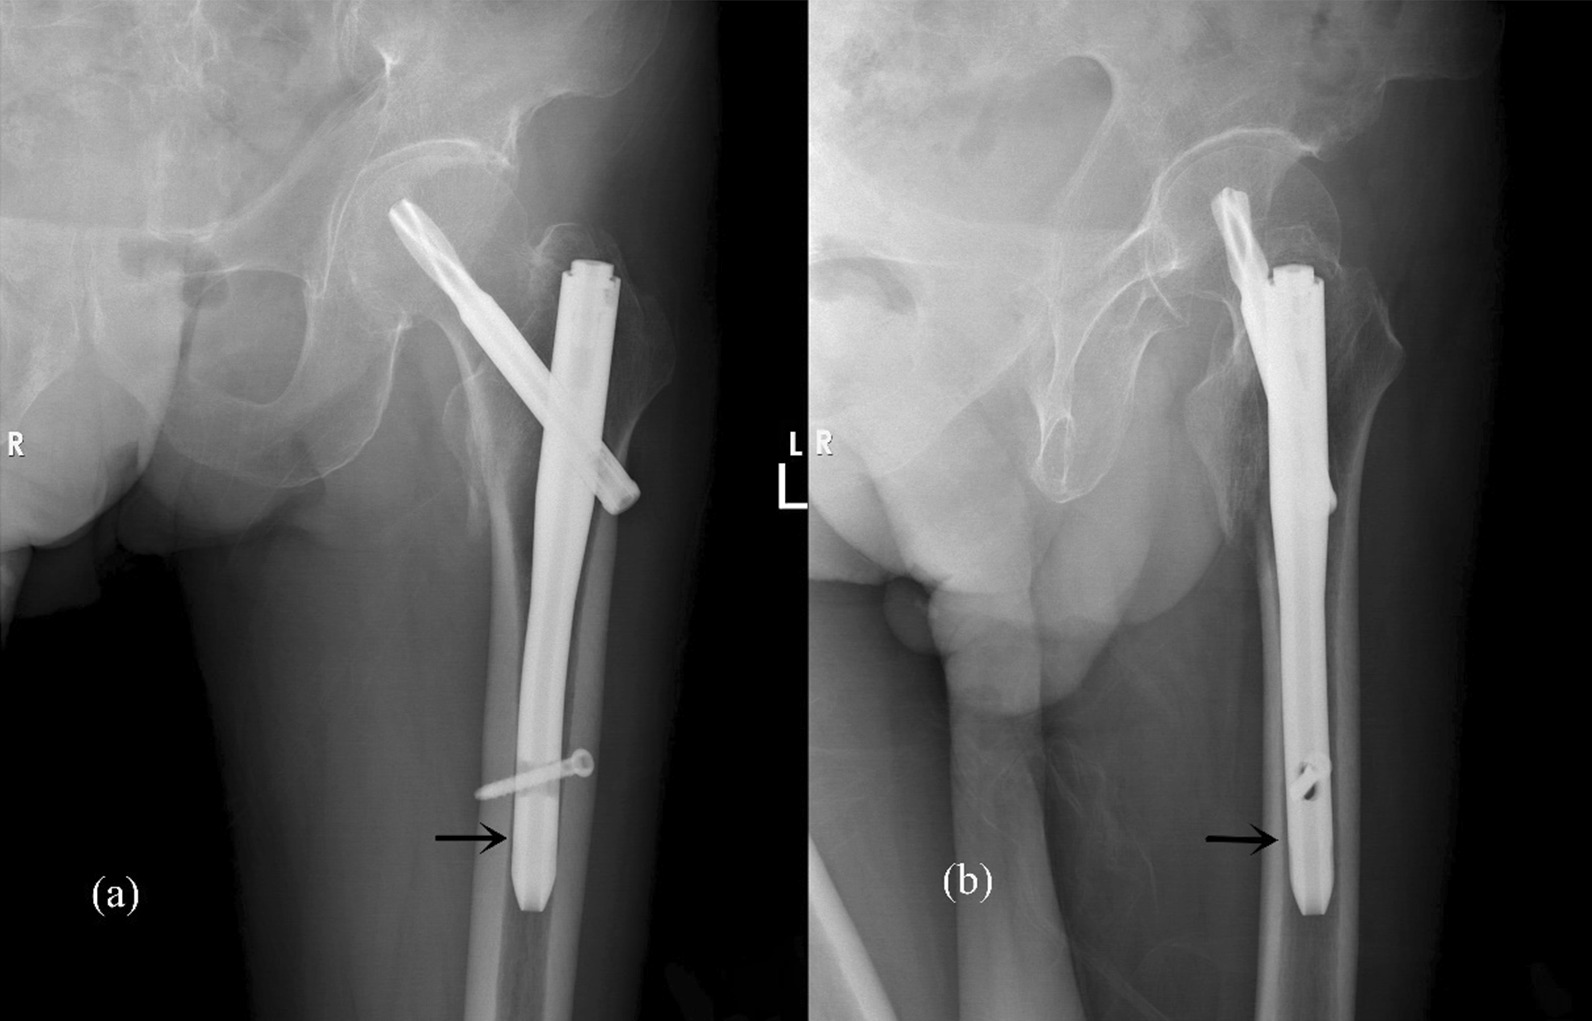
a AP radiograph. b Lateral radiograph. The diameter of femoral medullary cavity was measured at the layer where the medullary cavity was most fully filled with the distal nail.
